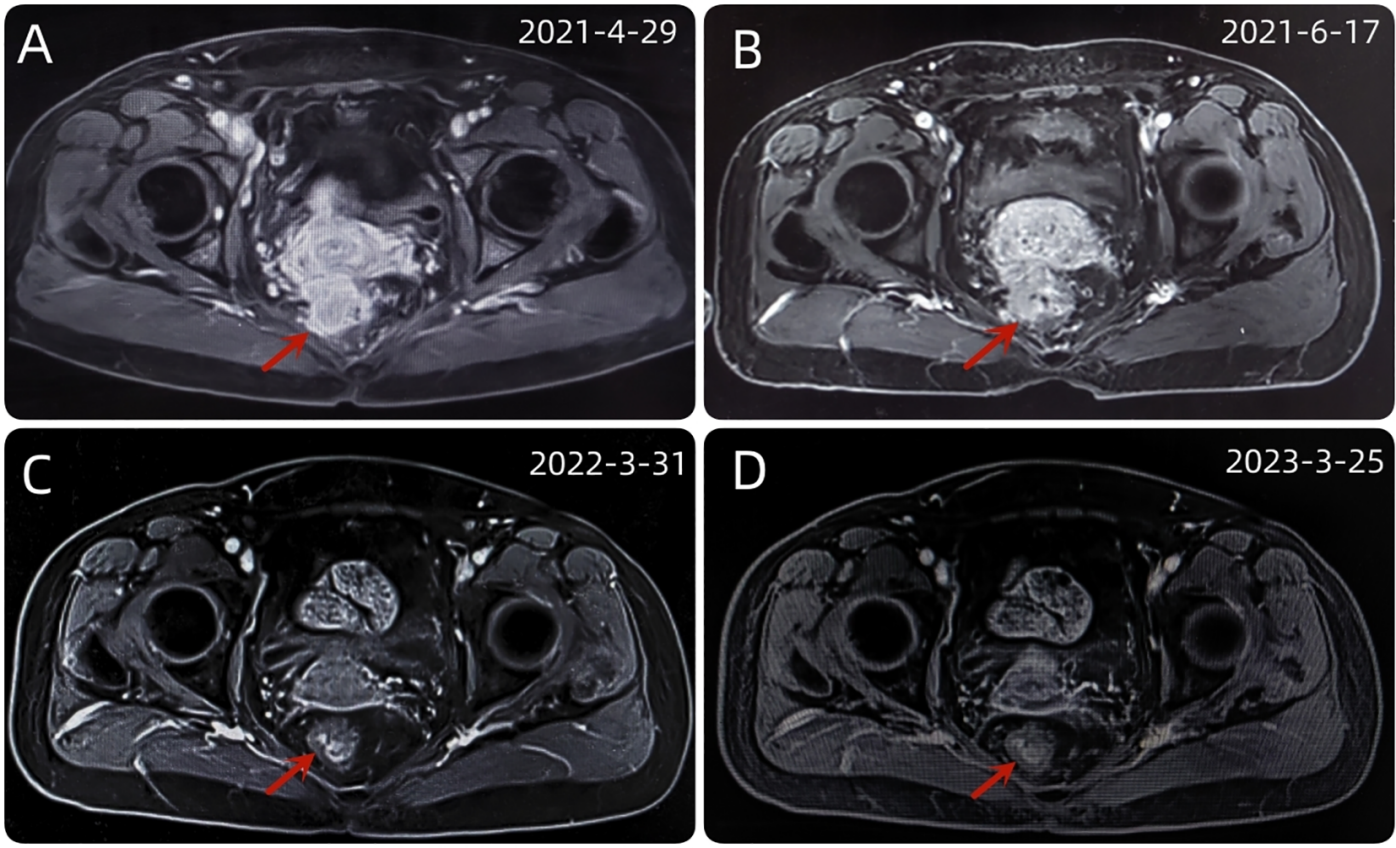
(A) The baseline abdominal MRI of the patient. (B) After 2 cycles of immunotherapy, the size and shape of the tumor did not change significantly. (C) After a series of comprehensive treatment, the original mass disappeared. (D) After 1 year of follow-up, the disease was stable and had no recurrence.
